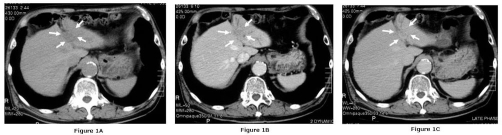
CT reveals a low-density mass (arrows) in the left lateral segment (A) with slight enhancement in the early phase (B) and further enhancement in the delayed phase (C).
